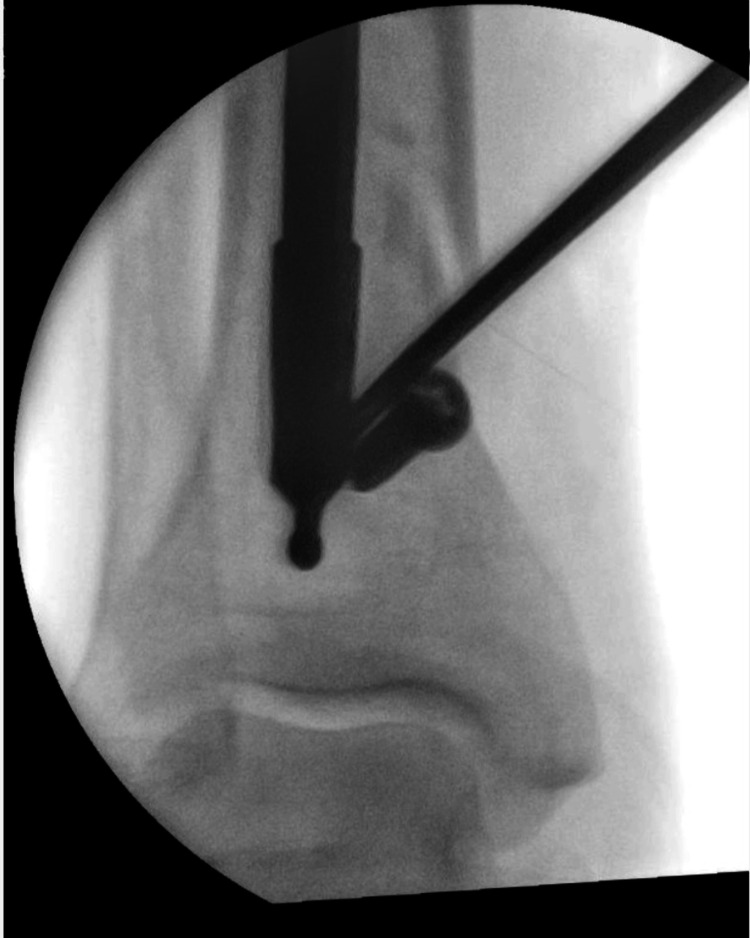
An X-ray demonstrating the nail being leveraged over the screw.
